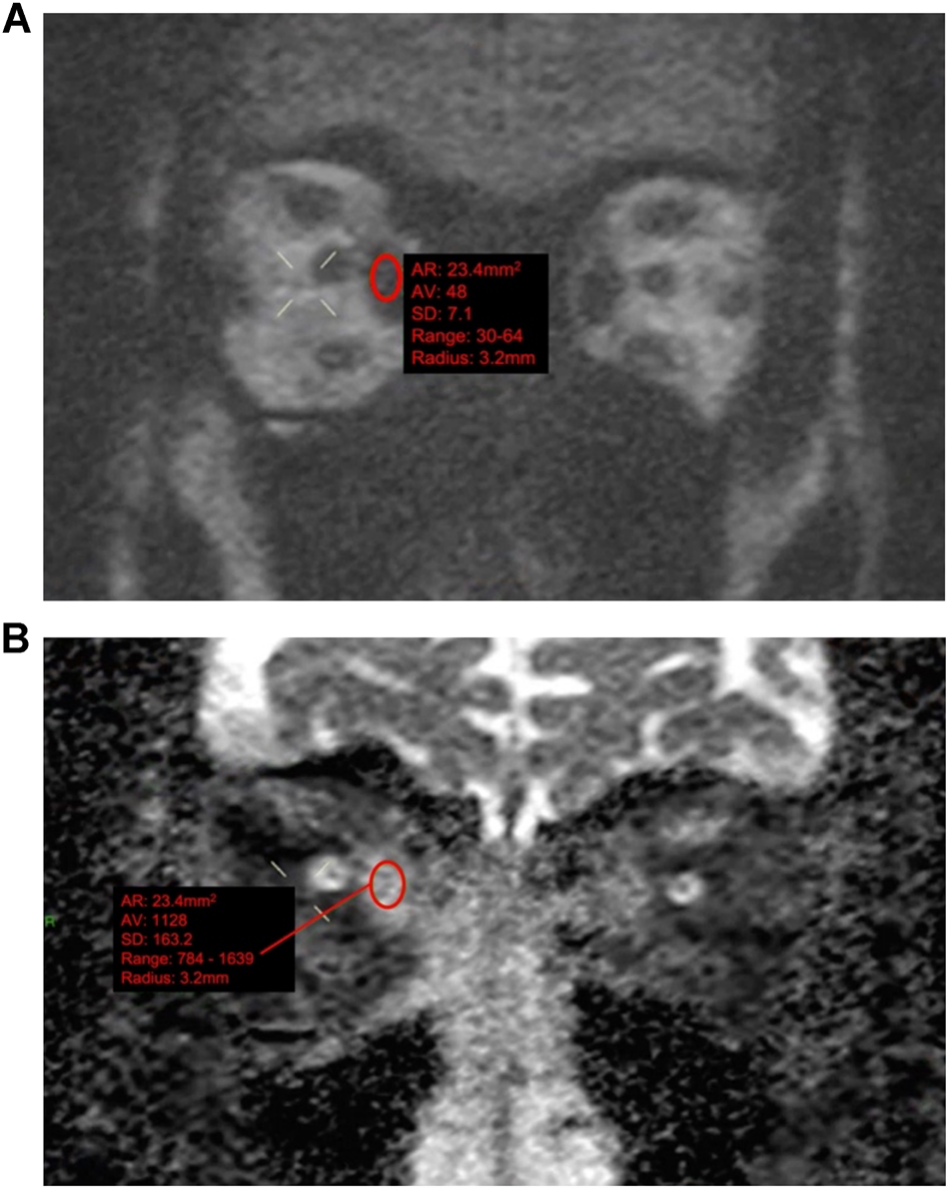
Representative examples of non-echoplanar diffusion-weighted imaging (non-EPI-DWI) of orbital EOMs in a patient with TED. Coronal orbital/EOM non-EPI-DWI MRI scan at a *b* value of 1000 (A) and apparent diffusion coefficient (ADC) image (B) show moderate-to-marked enlargement of the extraocular muscles and increased ADC values, notably at the right medial rectus (ADC value = 1128). Contour representing the freehand region-of-interest outline within the inner border of the active EOM
